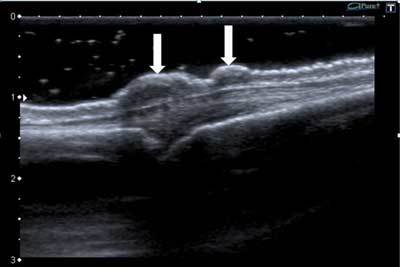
Axial T1-weighted image (WI) showed a hypointense nodule at the subcutaneous aspect of the extensor tendon of the fourth MCP joint. A subtler lesion was seen at of the extensor side of second MCP joint (Figure 3A, arrows).
